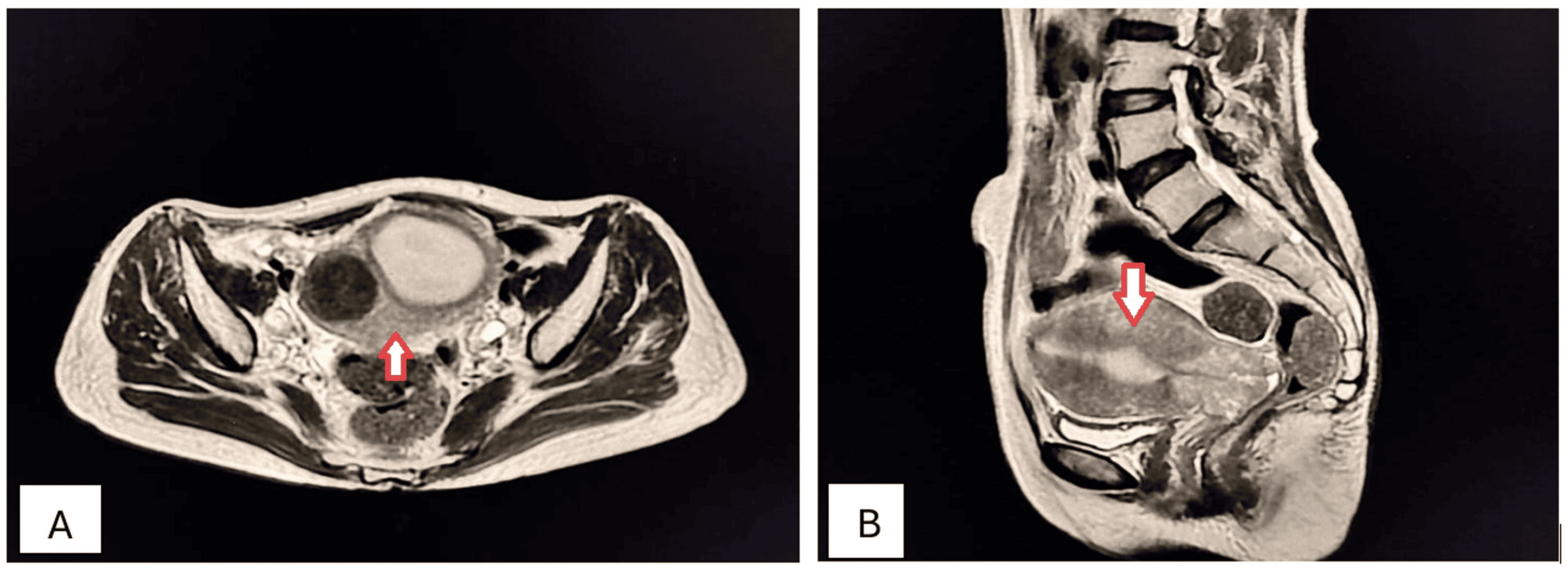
Adenomyosis of uterus (arrows) with cervical stenosis. Axial (A) and sagittal (B) T2W MRI shows an enlarged uterus with disproportionate enlargement of the posterior myometrium compared to the anterior myometrium, as well as numerous tiny cystic spaces in the transitional zone, consistent with adenomyosis. Similar changes are also observed in the cervix, consistent with cervical adenosis. A small, round, hypointense mass noted in the uterine myometrium on the right side is consistent with a fibroid. Adjacent to it on the left side, there is a well-circumscribed iso- to hyperintense endometrial collection due to cervical stenosis. T2W: T2-weighted
